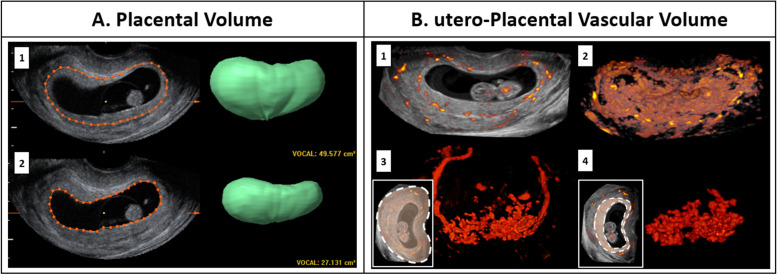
A: Measurement of placental volume (PV, cm^3^) using 3D ultrasound volumes and VOCAL measuring technique by subtracting 1. total pregnancy volume and 2. gestational sac volume. B: utero-placental vascular volume obtained by semi-automated measurements using three-dimensional power Doppler ultrasound images and Virtual Reality (VR) visualization. 1. Slice view of a complete 3D power Doppler vascular volume at 9 weeks GA, with surrounding grey values representing the uterine tissue. 2. Volume view of Fig. B1 showing the total obtained pregnancy volume, after setting a threshold for grey values. 3. Volume view of the complete 3D Power Doppler vascular volume. The anatomic orientation of the vasculature in color is demonstrated in the lower insert, delineated by a dashed white line. 4. Volume view of the utero-placental vascular volume (uPVV, cm^3^), calculated by subtracting uterine and fetal vascular volumes using a virtual brush to erase vascular voxels up to the myometrial-placental border, leaving the utero-placental vascular volume, delineated by the dashed with line
